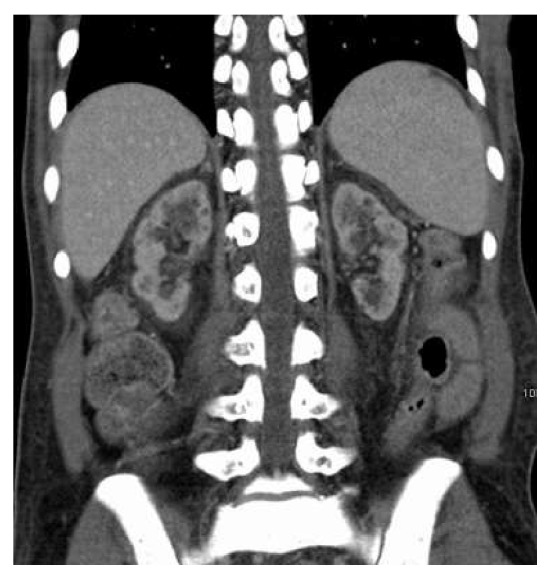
CT abdomen chronic cystic renal atrophy/chronic medical renal disease.
